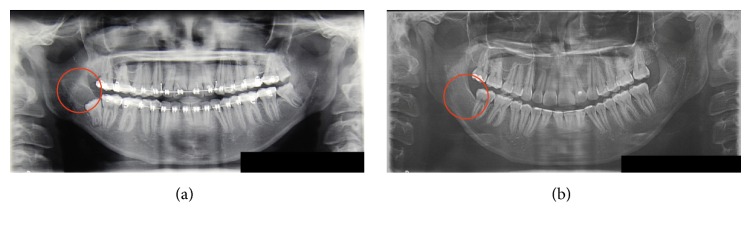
Panoramic radiograph before and after treatment. (a) Panoramic radiograph showed that the presence of a unilocular radiolucent lesion was shown in the right mandibular ramus, involving the impacted tooth 48. (b) The patient was followed up and, 12 months later, no sign of recurrence was detected.
